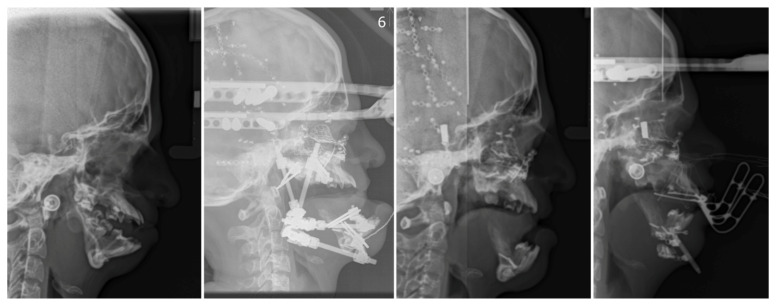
Cephalometric radiographs showing the basic steps of treatment related to the process of mandibular and maxillary osteodistraction aimed at improving facial contour and opening the upper airway.
